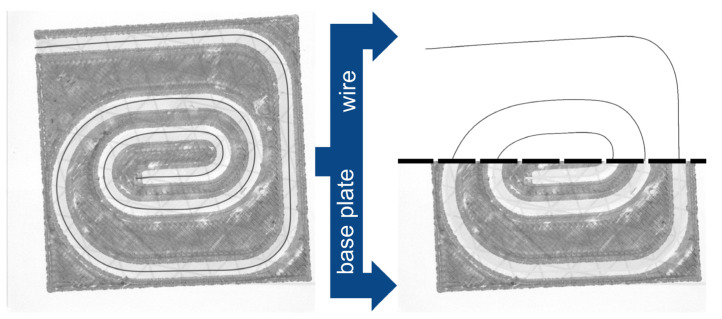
Separation of the CT image: The CT image (left) is separated, as shown by the black dashed line, into the wire (right top) and the base plate (right bottom) based on the color value of the pixels.
